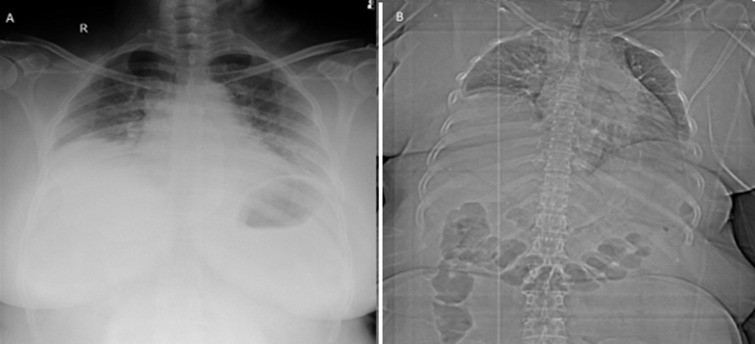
A) chest X-ray showing elevated right dome of diaphragm; B) computed tomography topogram of the patient showing eventration of right diaphragm
